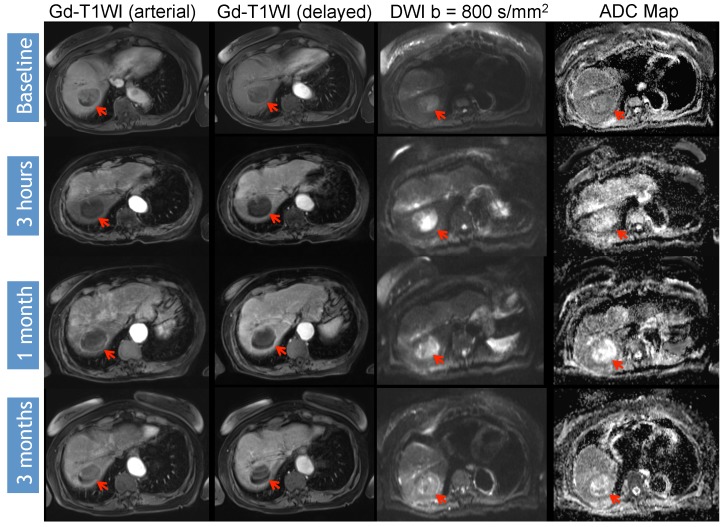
DWI (diffusion-weighted imaging) Response Assessment after DEB-TACE (drug-eluting bead transarterial chemoembolization). 65-year-old man presented with a segment VIII HCC (hepatocellular carcinoma) (arrow) without macrovascular invasion or extrahepatic disease. Note that there is no significant change in the post-treatment arterial Gadolinium (Gd)-enhancement pattern; although there is mild increase in the delayed Gd-enhancement over time. However, when analyzing DWI images, ADC (apparent diffusion coefficient) values increased progressively for ≥20% (baseline ADC 0.808 × 10^−3^ mm^2^/s, post-3 h ADC 1.60 × 10^−3^ mm^2^/s, post-one month ADC 2.30 × 10^−3^ mm^2^/s and post-three month ADC 2.80 × 10^−3^ mm^2^/s), and visually, the central area of restriction is no longer noted. The findings represent a subjective and objective measurement of response, in this case representing complete response (CR) to therapy.
